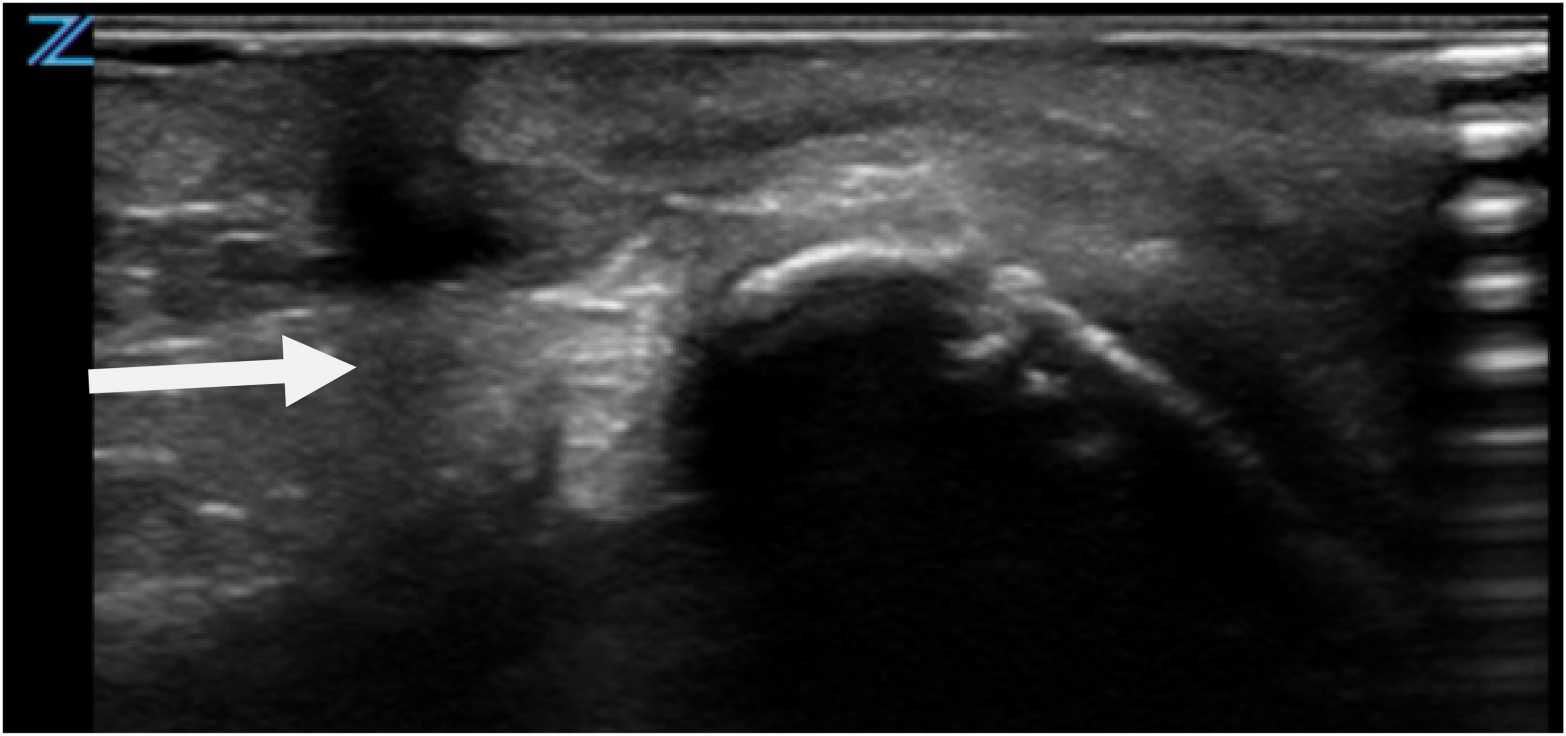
Ultrasonographic evaluation of CMCC. Imaging reveals a blind-ended tract extending caudally, with no evidence of deeper structural involvement.
